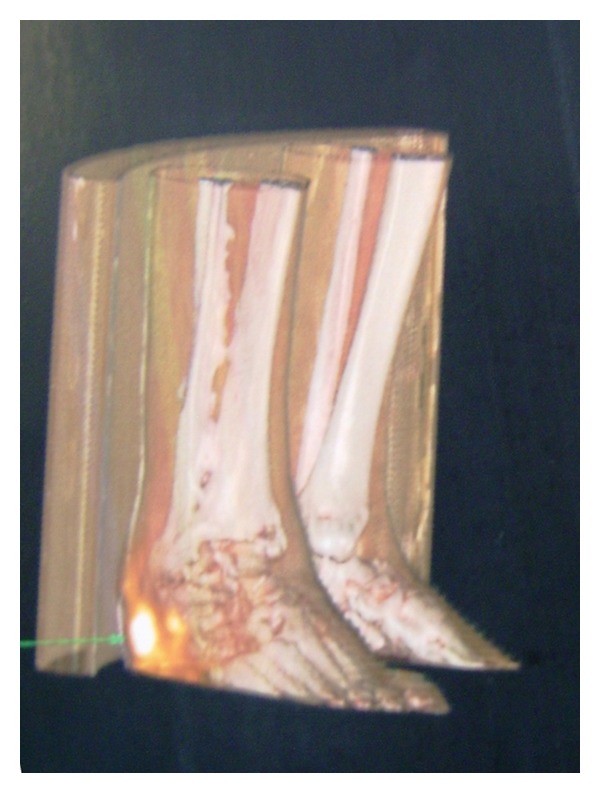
CT scan 3D of lower limb.
